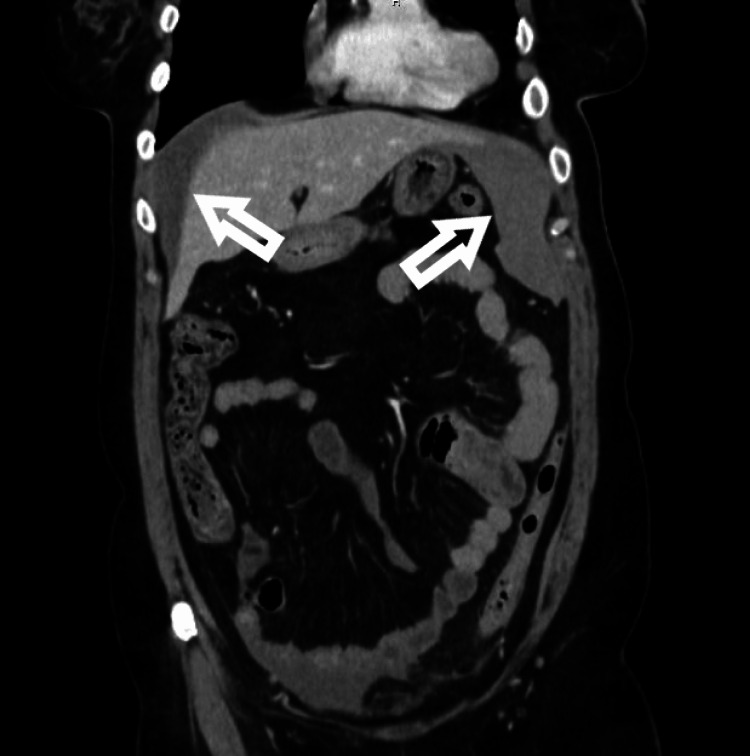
CT abdomen and pelvis with arrows pointing to free fluid in the abdomen indicating hemoperitoneum
